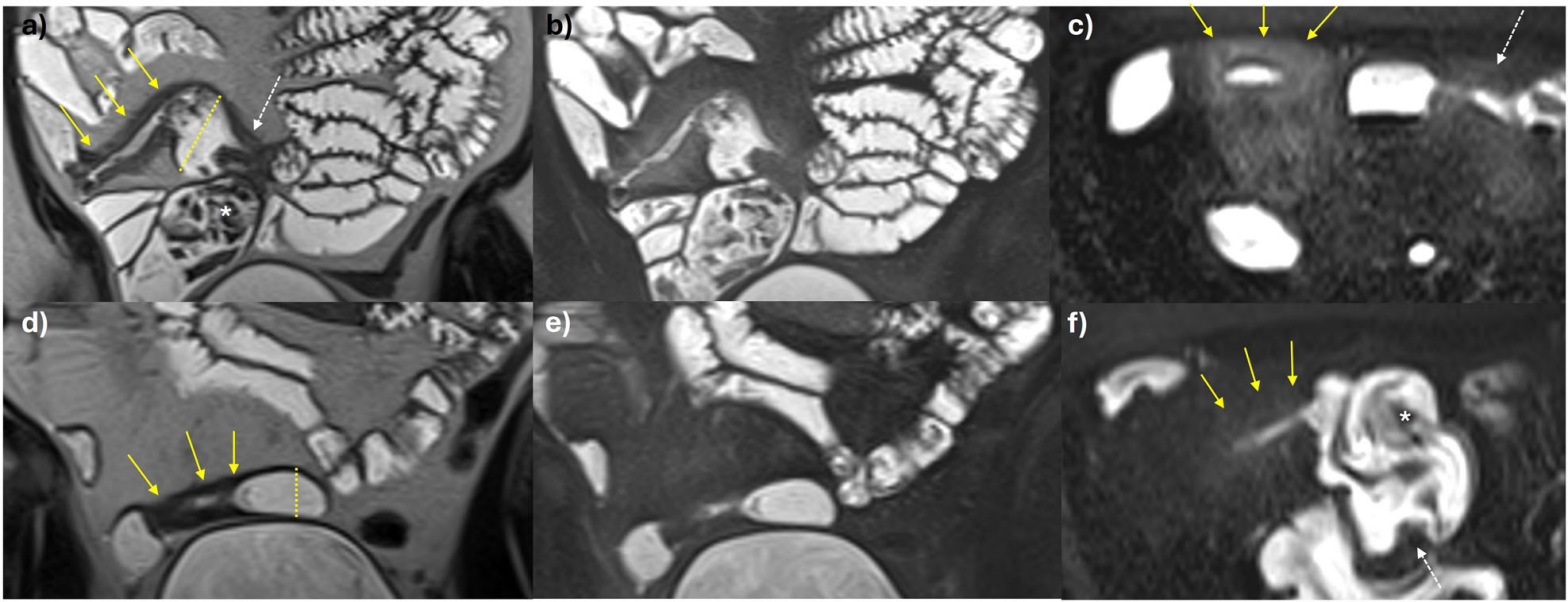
Treatment response assessment. 34-year-old female patient who stopped previous biologic treatment due to developing antibodies and presented with worsening diarrhoea. Baseline MRE study: a coronal T2-weighted image shows distal ileal disease with mural thickening (solid arrows) and perimural fat stranding with dilation of the immediately upstream loop of bowel (dashed line), indicating active, stricturing disease. Above this, there is a shorter stricture (dashed arrow) with upstream bowel dilation with enteroliths (asterisk). b Coronal and (c) axial T2 fat-saturated images show corresponding increased mural and perimural signal reflecting oedema in these same segments (solid and dashed arrows). The patient started Ustekinumab treatment, and a repeat MRE was performed 6 months after baseline to assess for response. d Coronal T2-weighted, (e) coronal, and (f) axial T2 fat-saturated images show significant transmural response. There is reduced mural and perimural T2 signal (oedema) shown in the longer segment of disease (arrows) and improved upstream dilation (dashed line). Although activity in the shorter segment of stricturing disease (dashed arrow) has improved, there remains proximal dilation mostly due to chronic disease (asterisk). MRE, MR enterography
